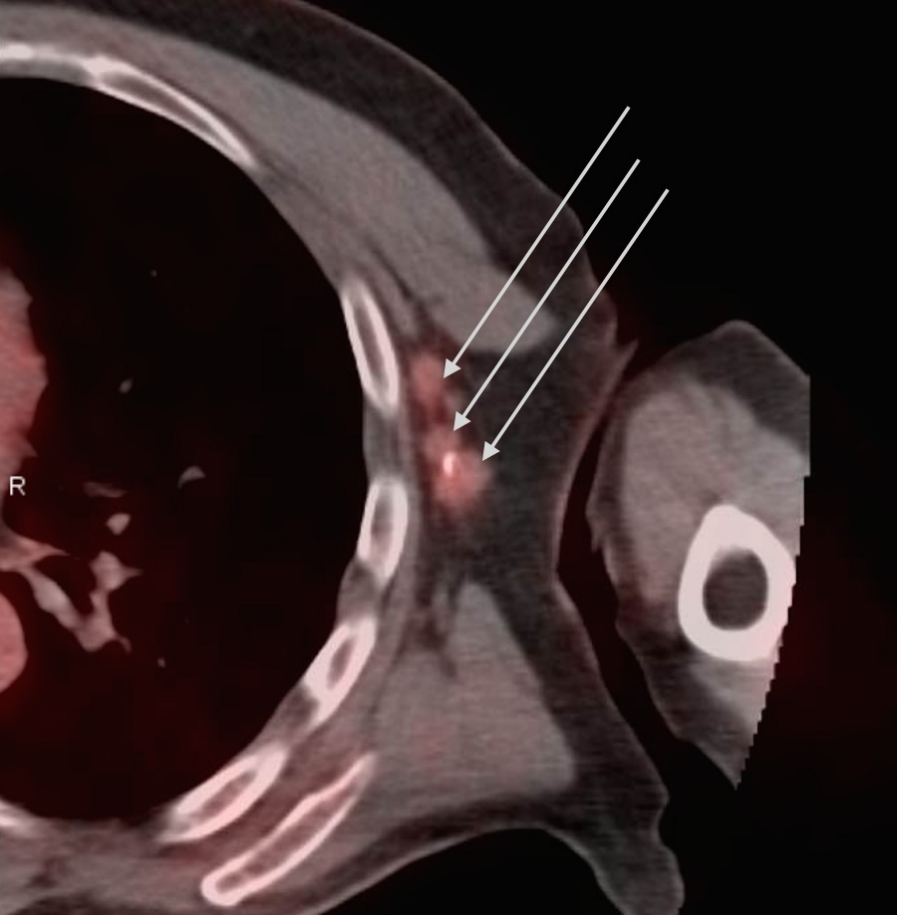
Positron emission tomography–computed tomography scan (PET-CT) showing a cluster of metabolically active left axillary lymph nodes measuring up to 1.5 cm
